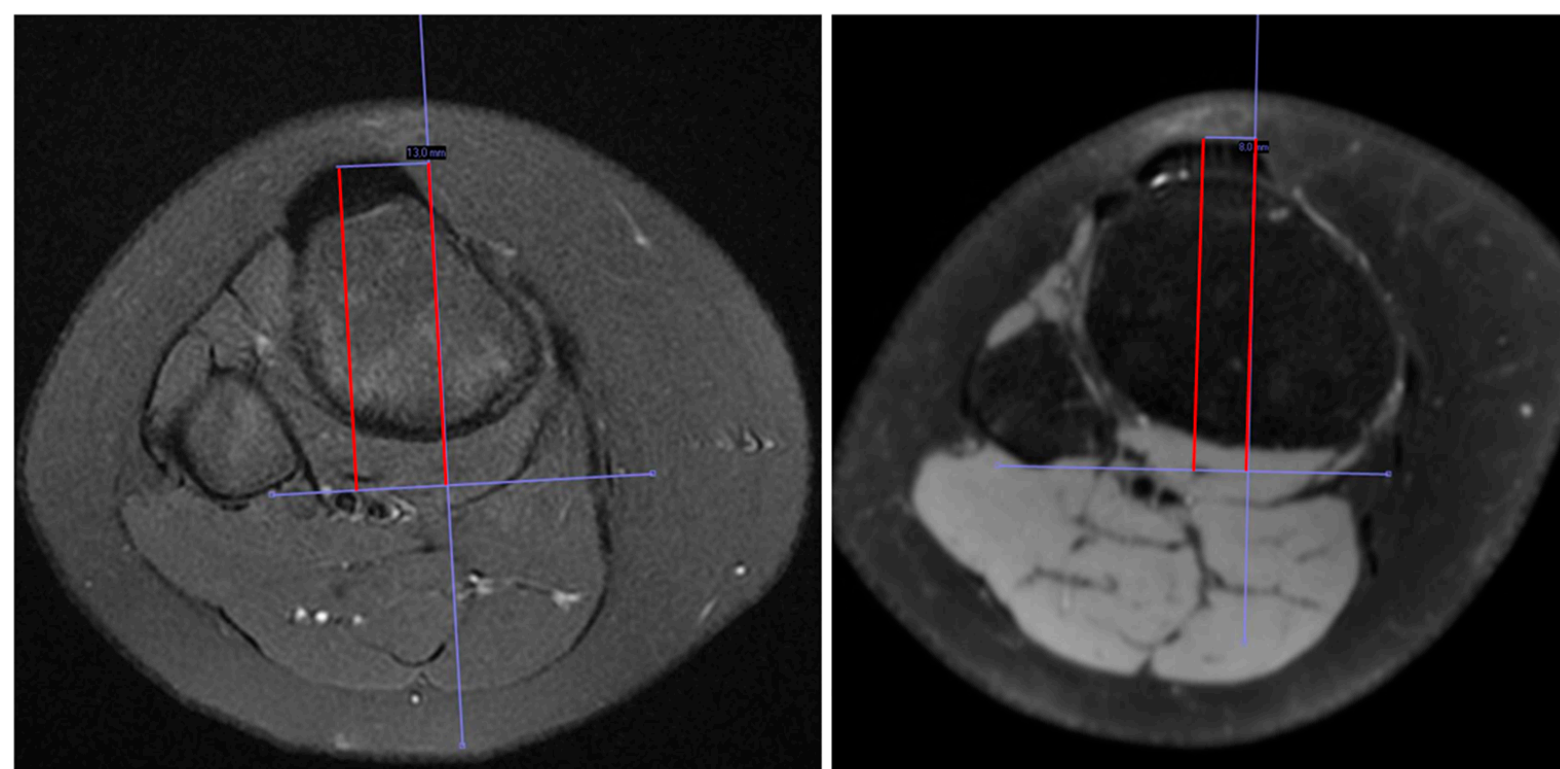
Magnetic Resonance Imaging (MRI) at baseline (left) and after 3 years (right) in a patient aged 10 years at the time of diagnosis demonstrated a reduction in the Tibial Tuberosity–Trochlear Groove (TT-TG) distance (the distance between the red lines).
